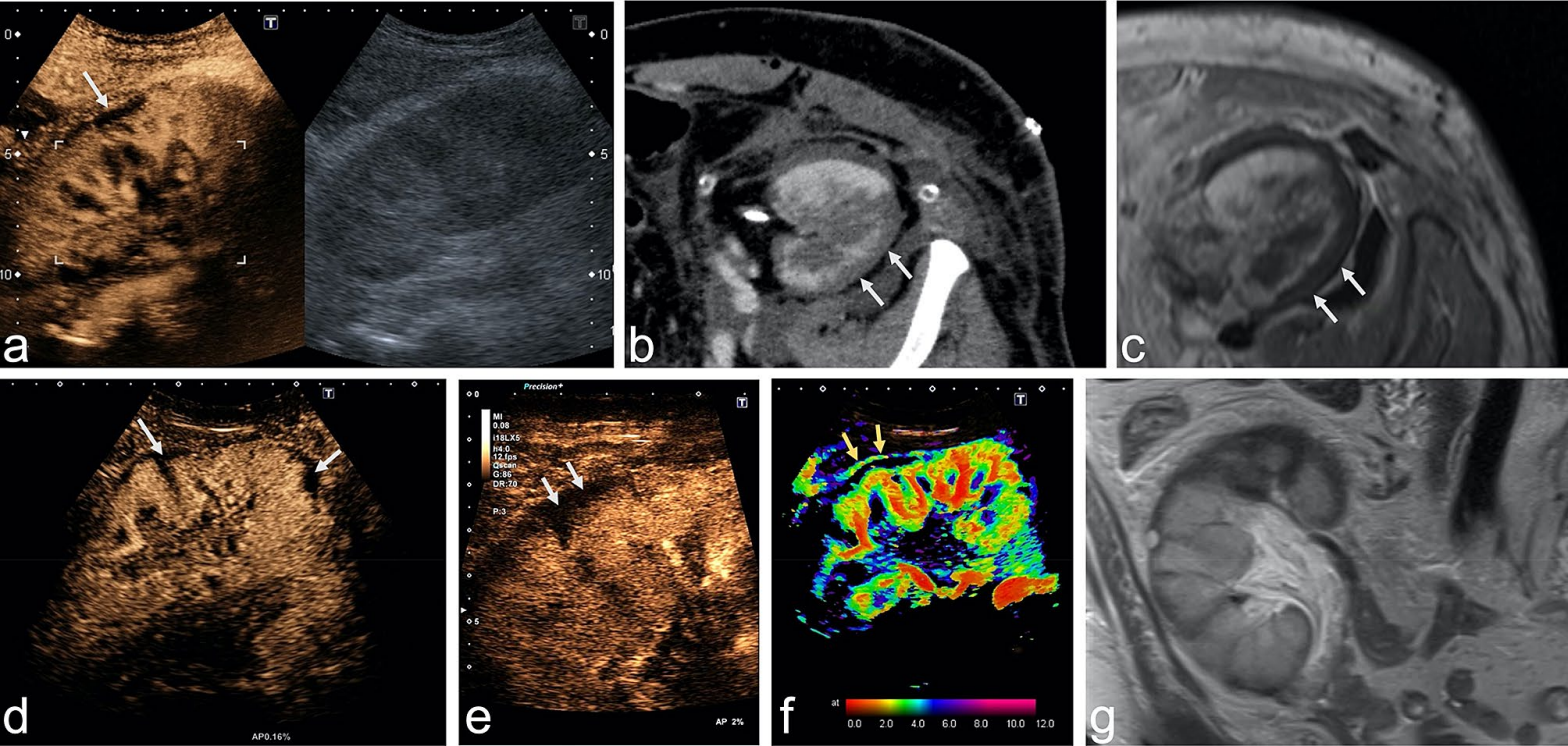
Comparison of modalities in the examination of a kidney transplant with suspected RCN. Upper row: Case showing partial RCN in a renal transplant in a 51-year-old man with end-stage renal failure due to shrunken native kidneys. (a) CEUS (0:59 min) and B-mode US of the renal transplant showing typical reverse rim sign with partial loss of enhancement in subcapsular cortex. (b,c) CeCT (b) and ceMRI (c) showing the hypoenhanced rim of the renal cortex in the transverse plane. Lower row: A case of RCN in a 54-year-old woman with renal transplant. (d) CEUS (1:13 min) using a convex multifrequency probe clearly identifies the reverse rim sign as a nonenhanced rim in the superficial cortex and lower pole (arrows). (e) Increased spatial resolution by use of a linear probe, which is important for second look if CEUS with convex probe shows inconclusive findings. (f) Parametric arrival time imaging of CEUS depicts arterial inflow in interlobar arteries and cortex within 4 s (red and green). Arrows indicate the so-called cortical rim sign with preserved blood supply by capsular arteries (arrows). (g) Coronal T2w MR image confirms the cortical structure defect and moreover shows cortical perfusion deficit at the upper pole of the renal transplant. RCN denotes renal cortical necrosis; CEUS, contrast-enhanced ultrasound; ceCT, contrast-enhanced computed tomography; ceMRI, contrast-enhanced magnetic resonance imaging.
